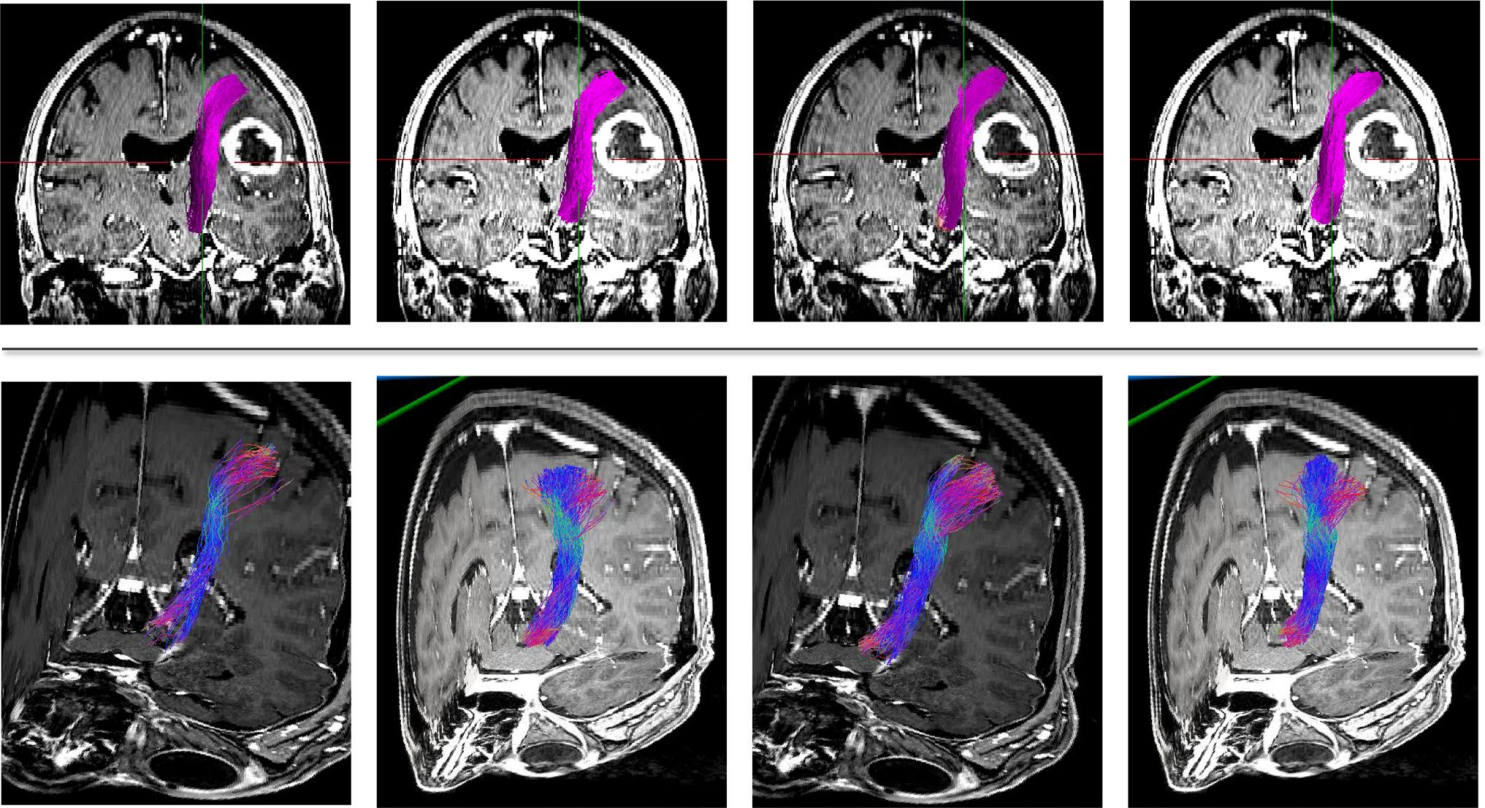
MRI T1-weighted + gadolinium in coronal view depicting the CST as reconstructed by Rater 1. Upper line: patient with non-contrast enhancing fronto-temporo-insular glioma left. From left to right: DTI-FT round 1, DTI-FT round 2. QBI-FT round 1, QBI-FT round 2. Lower line: patient with contrast enhancing glioma left frontal. From left to right: DTI-FT round 1, DTI-FT round 2. QBI-FT round 1, QBI-FT round 2. (MRI, magnetic resonance imaging; CST, Corticospinal Tract; QBI, Q-ball Imaging; DTI, Diffusion Tensor Imaging)
